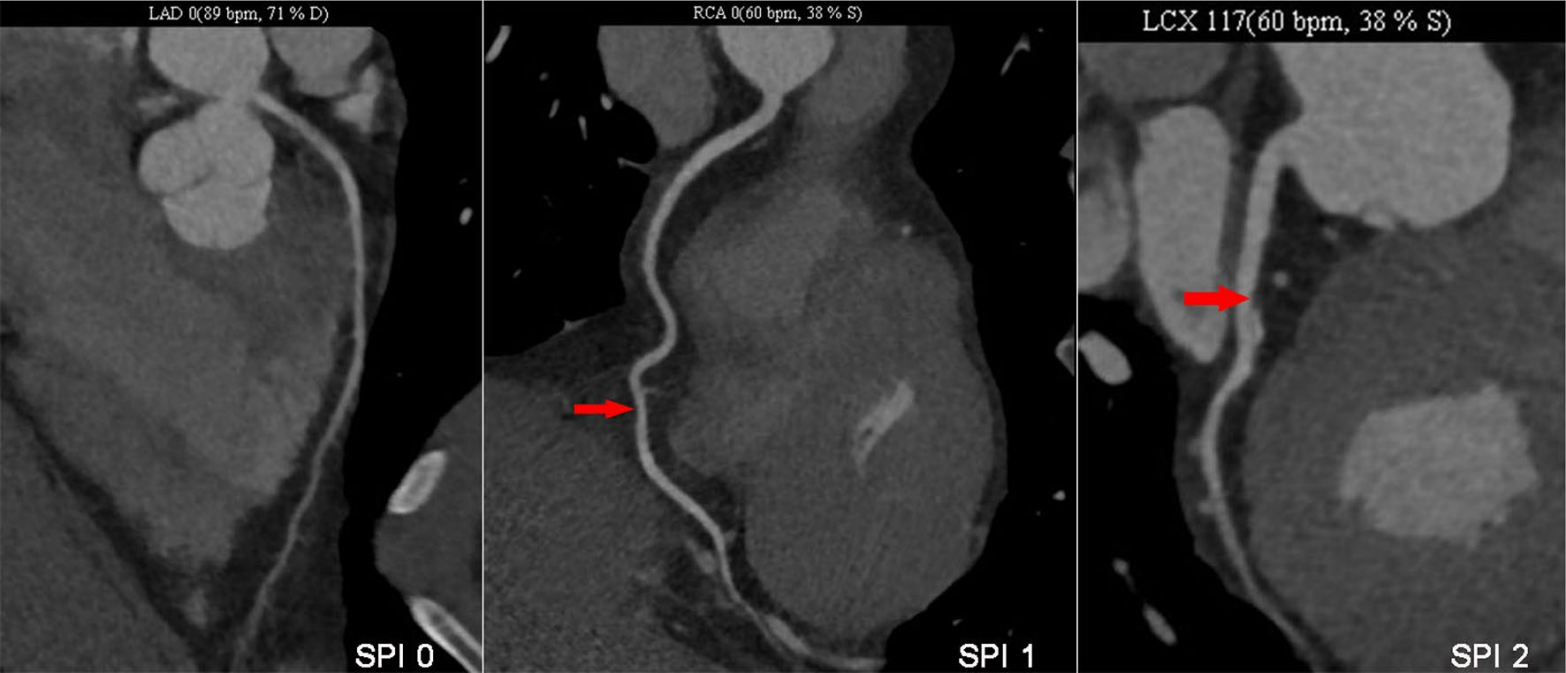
SPI classification assessed by coronary CTA.
